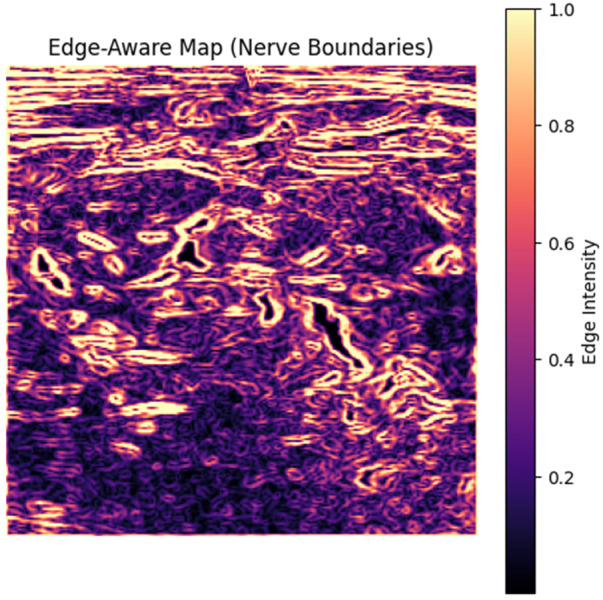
Edge-aware boundary map derived from the denoised ultrasound image using Sobel operators for gradient calculation, emphasizing nerve boundaries and anatomical transitions.
